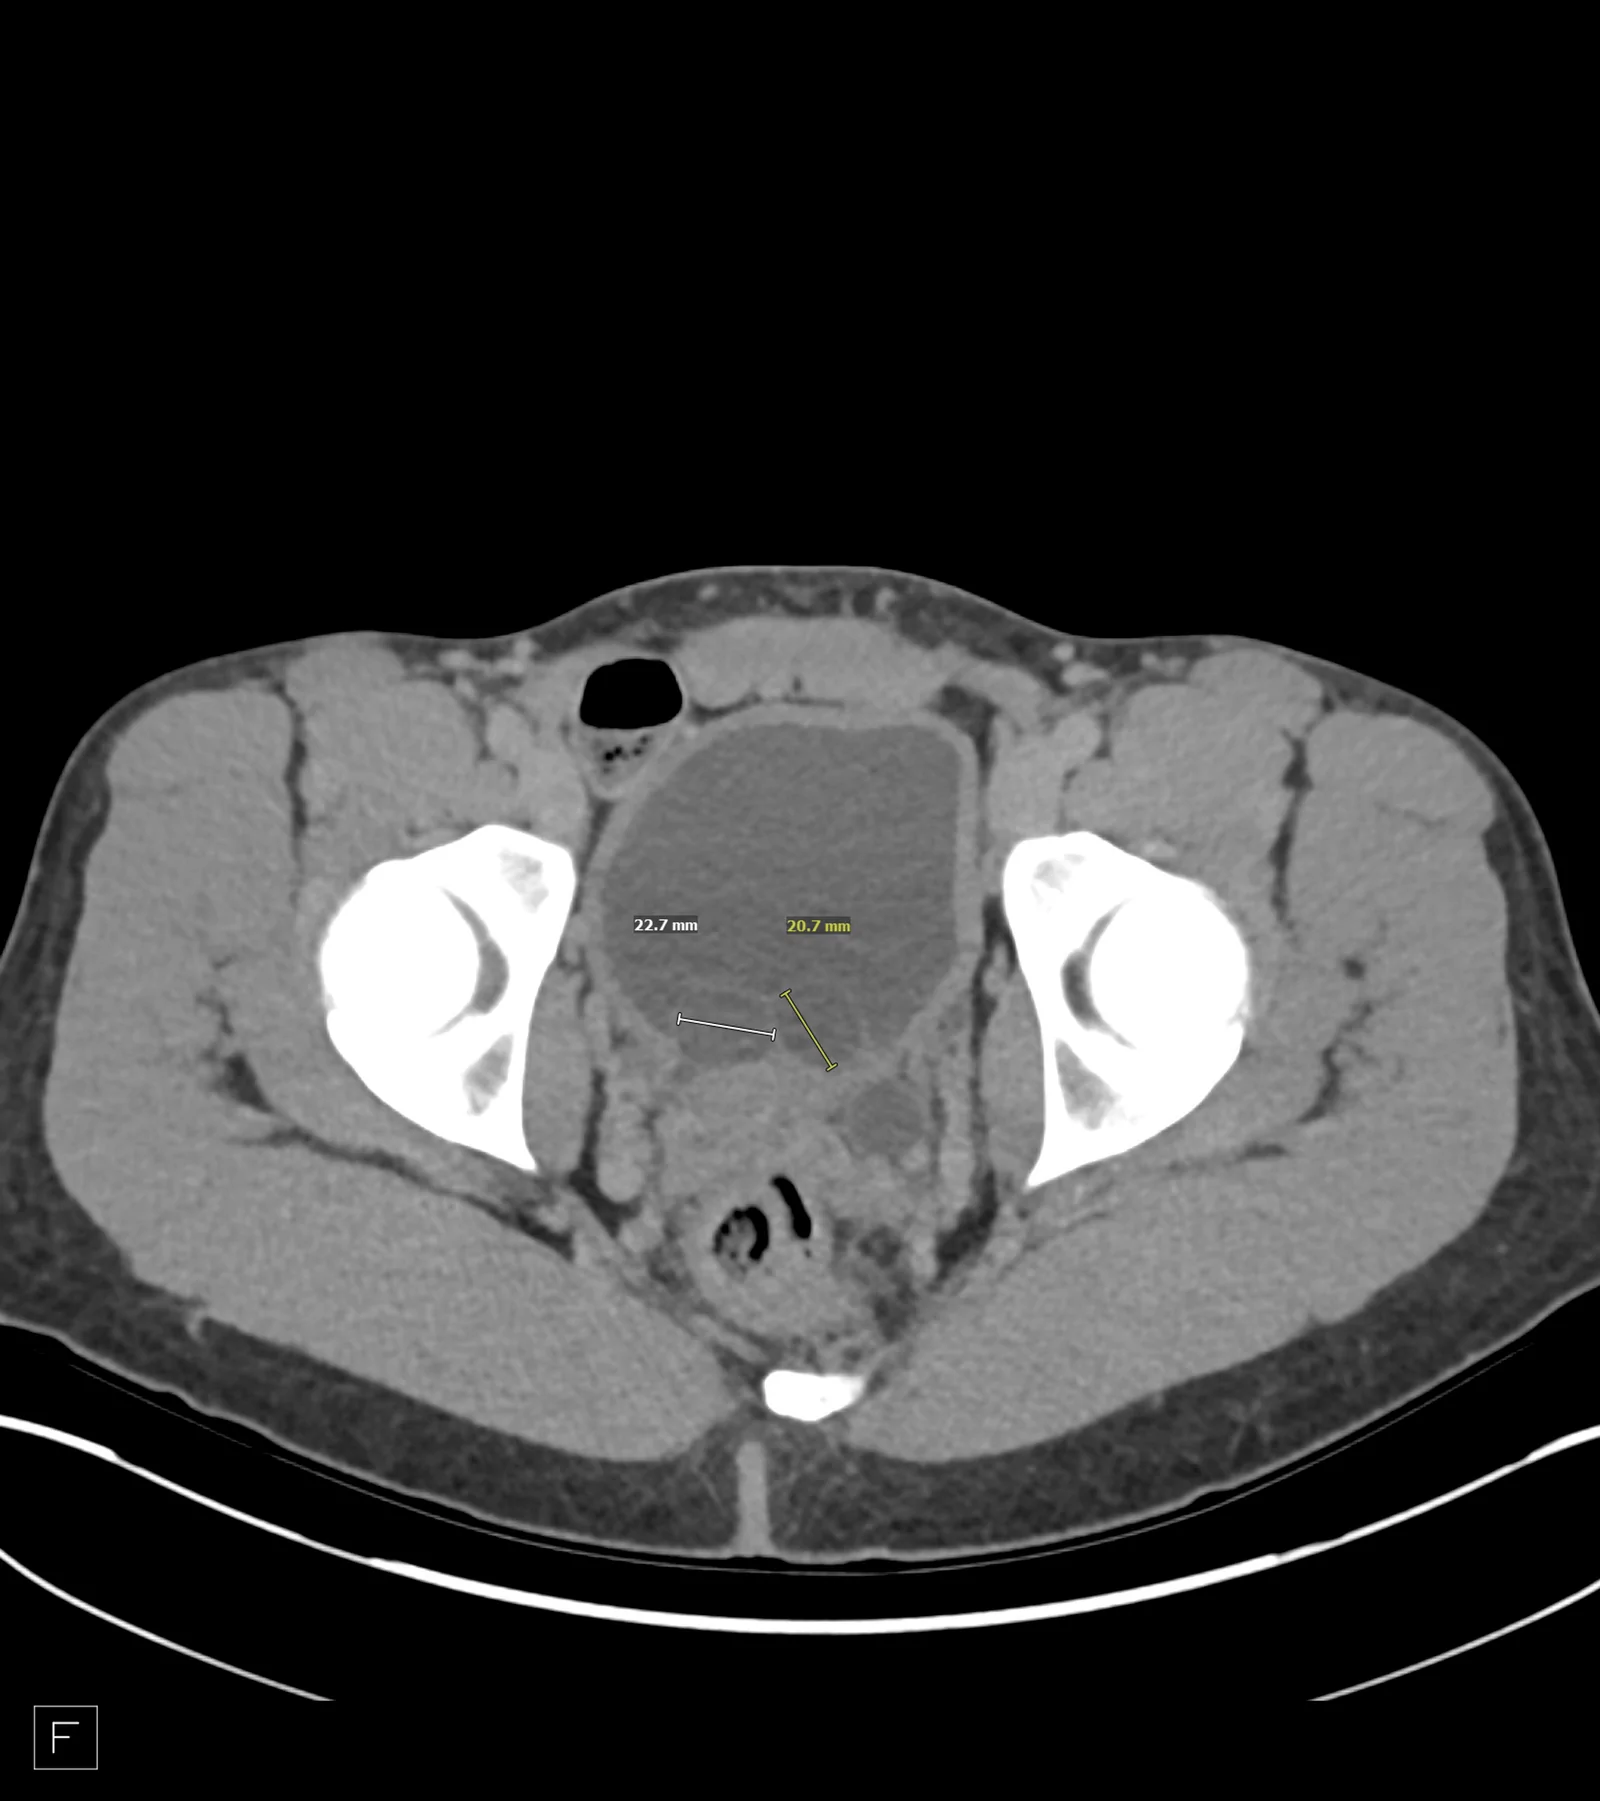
CT urography demonstrating bilateral intravesical ureteroceles at the distal ureters within the bladder.
